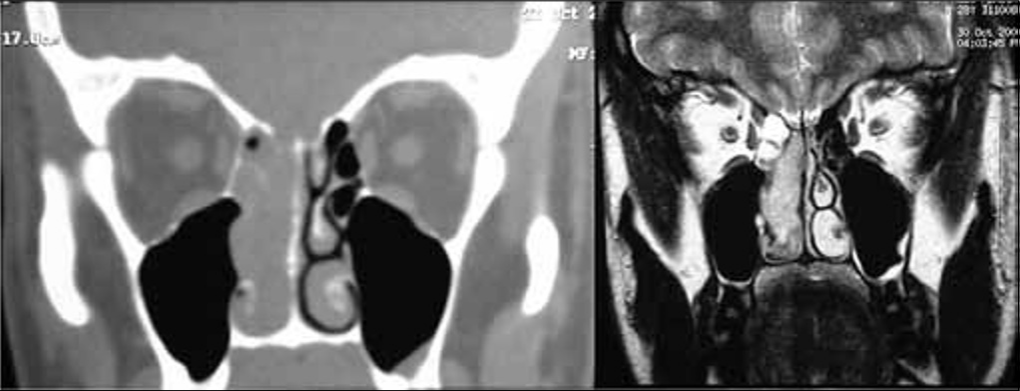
Preoperative CT and MRI of the patient JPP; stage Kadish D and Dulguerov T2n1.
